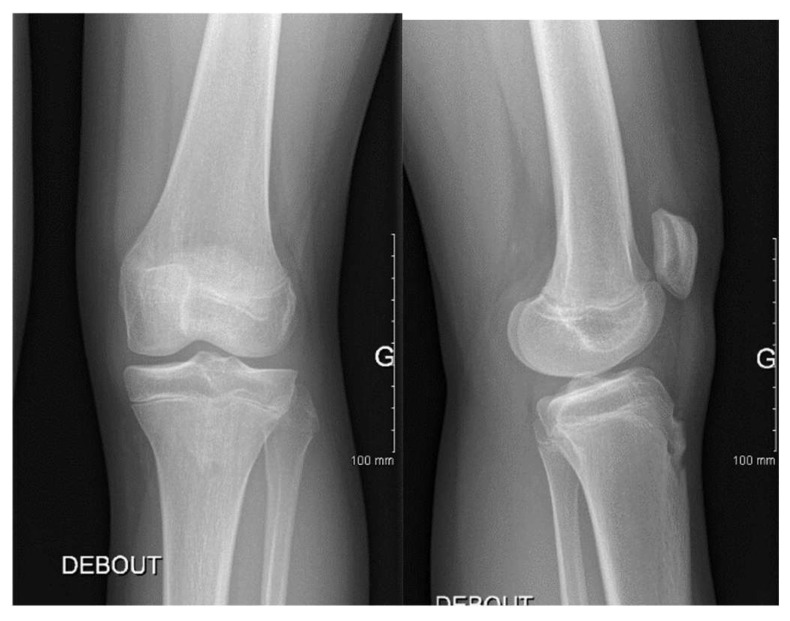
Patient S.C.S. (13 years old). Anteroposterior and lateral knee radiographs of a patient diagnosed with medial discoid meniscus. The standing anteroposterior (left) and lateral (right) X-ray views showed no obvious bony abnormalities. The joint space appeared to be preserved, with no signs of degenerative changes or malalignment.
